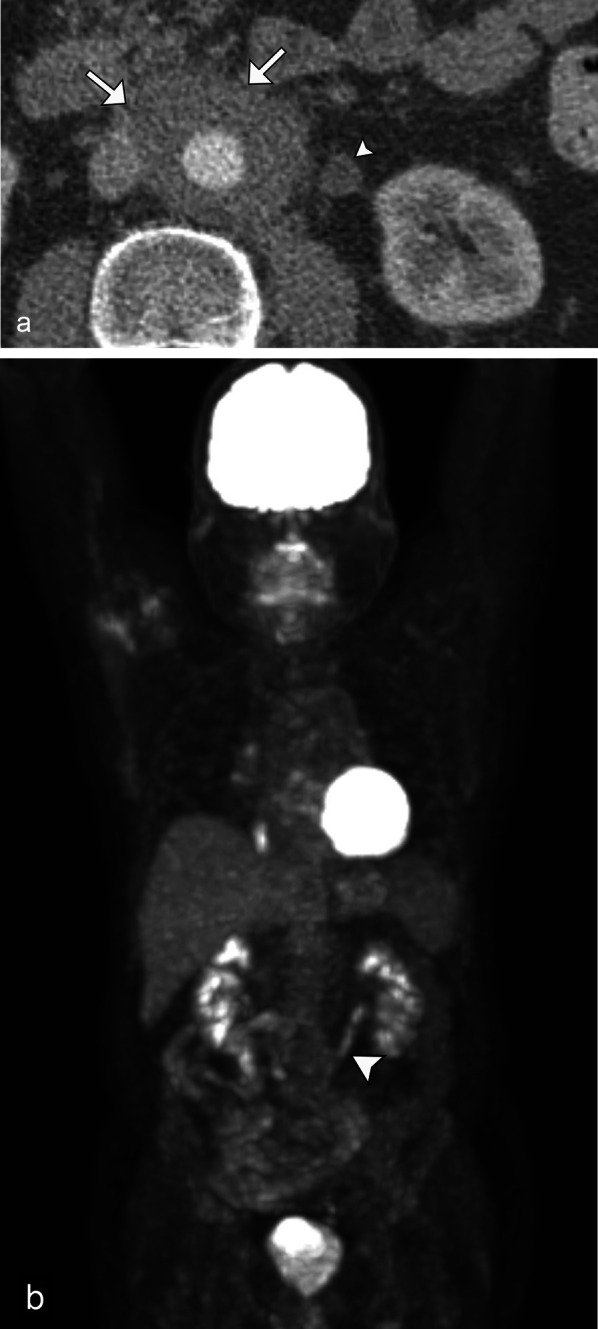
Retroperitoneal fibrosis.* Axial contrast CT (a) of the abdomen demonstrates retroperitoneal mass-like soft thickening enveloping the aorta without lifting it anteriorly (arrows), and a dilated left ureter (arrowhead). This mimics retroperitoneal lymphomatous disease, although lymphoma tends to lift/displace the aorta/IVC. F18-FGD PET/CT MIP (b) demonstrates a medialized left ureter (arrow). Note the lack of FDG avidity in the expected region of the retroperitoneal mass. Findings are consistent with retroperitoneal fibrosis
